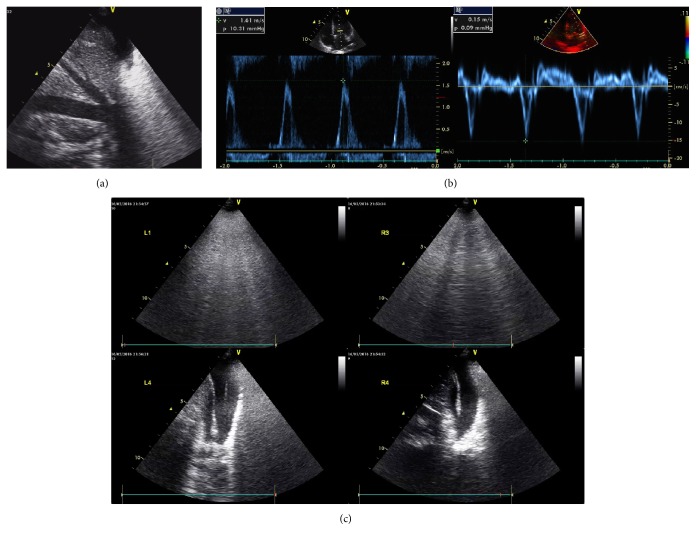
Abnormal findings of the Focused Ultrasound Assessment on admission of case 1. (a) IVC exam. Fixed and enlarged IVC and hepatic vein represented no fluid responsiveness and maybe hypervolemia. (b) Assessment of diastole. Restrictive diastolic dysfunction was presented and PAOP estimated by E/e' was increased. (c) Lung ultrasound exam. Bilateral multiple B lines, with posterior atelectasis and plural effusion, indicate diffuse sonographic interstitial syndrome.
